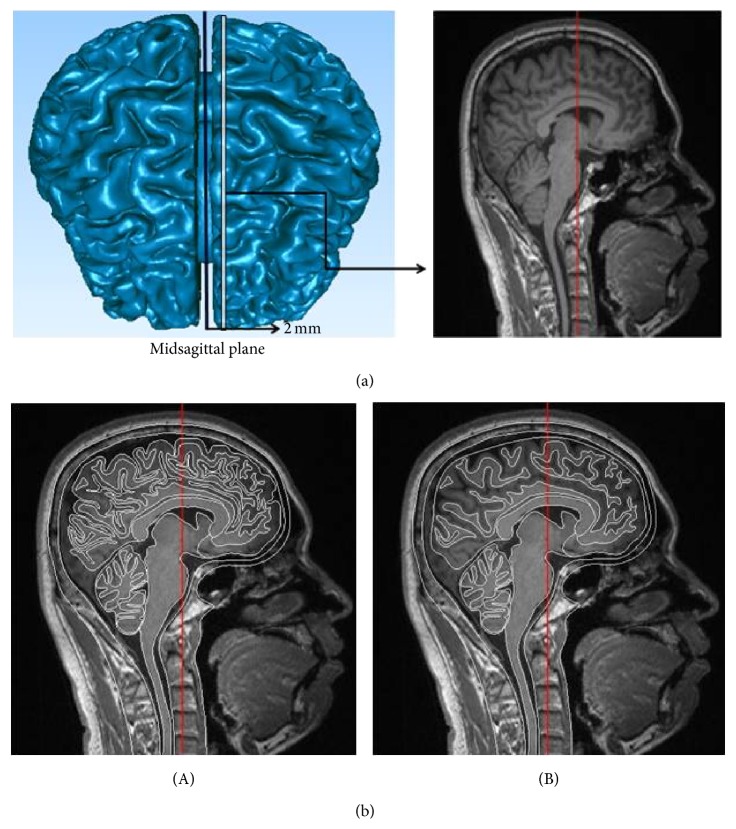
(a) The chosen slice position and parasagittal MRI data. (b) The extracted geometric profile of the chosen parasagittal MRI data using CATIA. (A) The geometric profile with gyri and sulci. (B) The geometric profile without gyri and sulci.
